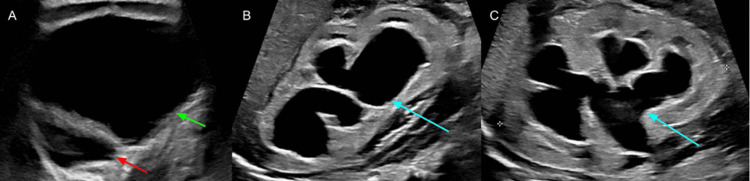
Renal and bladder ultrasound at presentation (A) Transverse view demonstrating a distended bladder (green arrow) and bladder diverticulum (red arrow). (B) Sagittal view of the right kidney demonstrating hydronephrosis (blue arrow). (C) Sagittal view of the left kidney demonstrating hydronephrosis (blue arrow).
